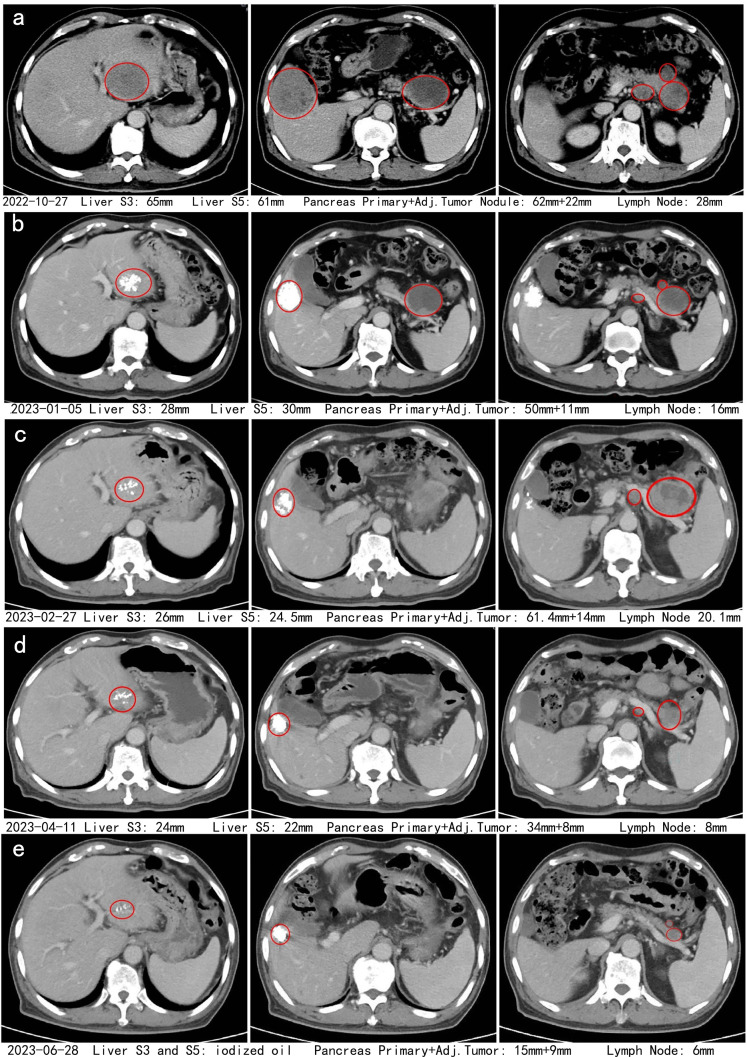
Serial abdominal computed omography (CT) images (axial view) during treatment. Red circles indicate the target lesions. For each of the five key time points (a–e), representative CT images at three consecutive anatomical levels are displayed, with the examination dates annotated. (a) Baseline (October 2022): A solid mass is seen in the pancreatic tail (yellow arrow, approximately 62 × 43 mm), with an adjacent cystic-solid tumor nodule located anterosuperiorly (related to the primary tumor, approximately 22 × 18 mm). Liver metastases are present in segments S3 and S5 (approximately 61 × 61 mm and 65 × 60 mm, respectively). Enlarged metastatic lymph nodes are noted inferior to the pancreatic body (approximately 28 × 18 mm). (b) Partial Response (January 2023): After first−line therapy (TACE plus gemcitabine/nab−paclitaxel), the primary pancreatic tail lesion, the adjacent cystic-solid tumor nodule, liver metastases (with iodized oil deposits), and metastatic lymph nodes all showed significant reduction in size. (c) Progressive Disease (February 2023): The primary pancreatic tail lesion (yellow arrow) and abdominal metastatic lymph nodes increased in size, consistent with disease progression (Table 1); the liver metastases (areas of iodized oil deposition) decreased in extent compared with prior imaging. (d) Marked Response (April 2023): After two cycles of cadonilimab combination chemotherapy, the primary pancreatic tail lesion, the adjacent cystic lesion, liver metastases (predominantly iodized oil deposits with further reduction in extent), and metastatic lymph nodes all demonstrated marked shrinkage, achieving a partial response (Table 1). (e) Near−Complete Response (June 2023): Following discontinuation of cadonilimab and completion of two subsequent cycles of chemotherapy, the enhancing lesions in the pancreas and lymph nodes were significantly reduced, with only stable iodized oil deposits remaining in the liver, corresponding to a near−complete response (Table 1).
